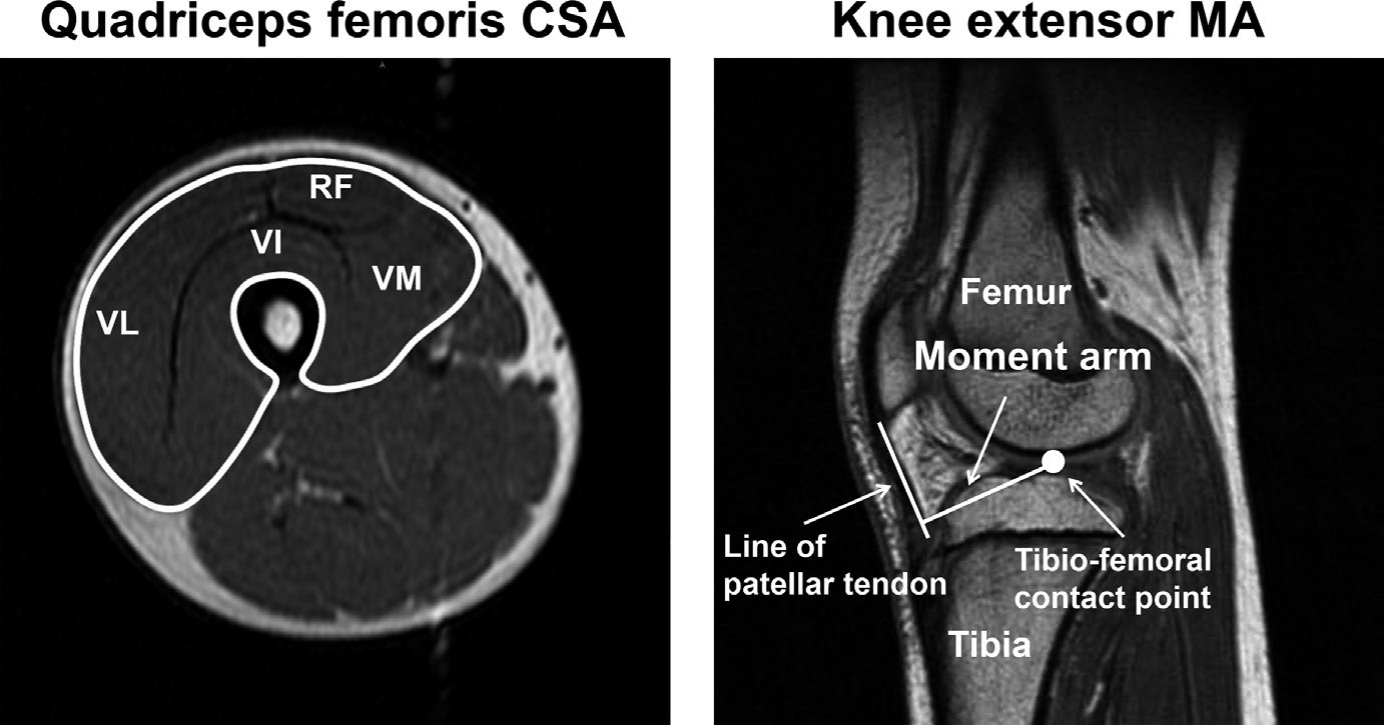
Representative magnetic resonance imaging scans for measuring the quadriceps femoris muscle volume (MV) and knee extensor moment arm (MA). The mid‐thigh quadriceps femoris cross‐sectional are (CSA) obtained at 50% of the thigh length. The quadriceps femoris muscle included rectus femoris (RF), vastus intermedius (VI), vastus lateralis (VL), and vastus medialis (VM). The quadriceps femoris MV was calculated by multiplying the sum of the CSAs along their length at intervals of 10 mm. The knee extensor MA was calculated as the distance between tibio‐femoral contact point and mid‐line of the patellar tendon
